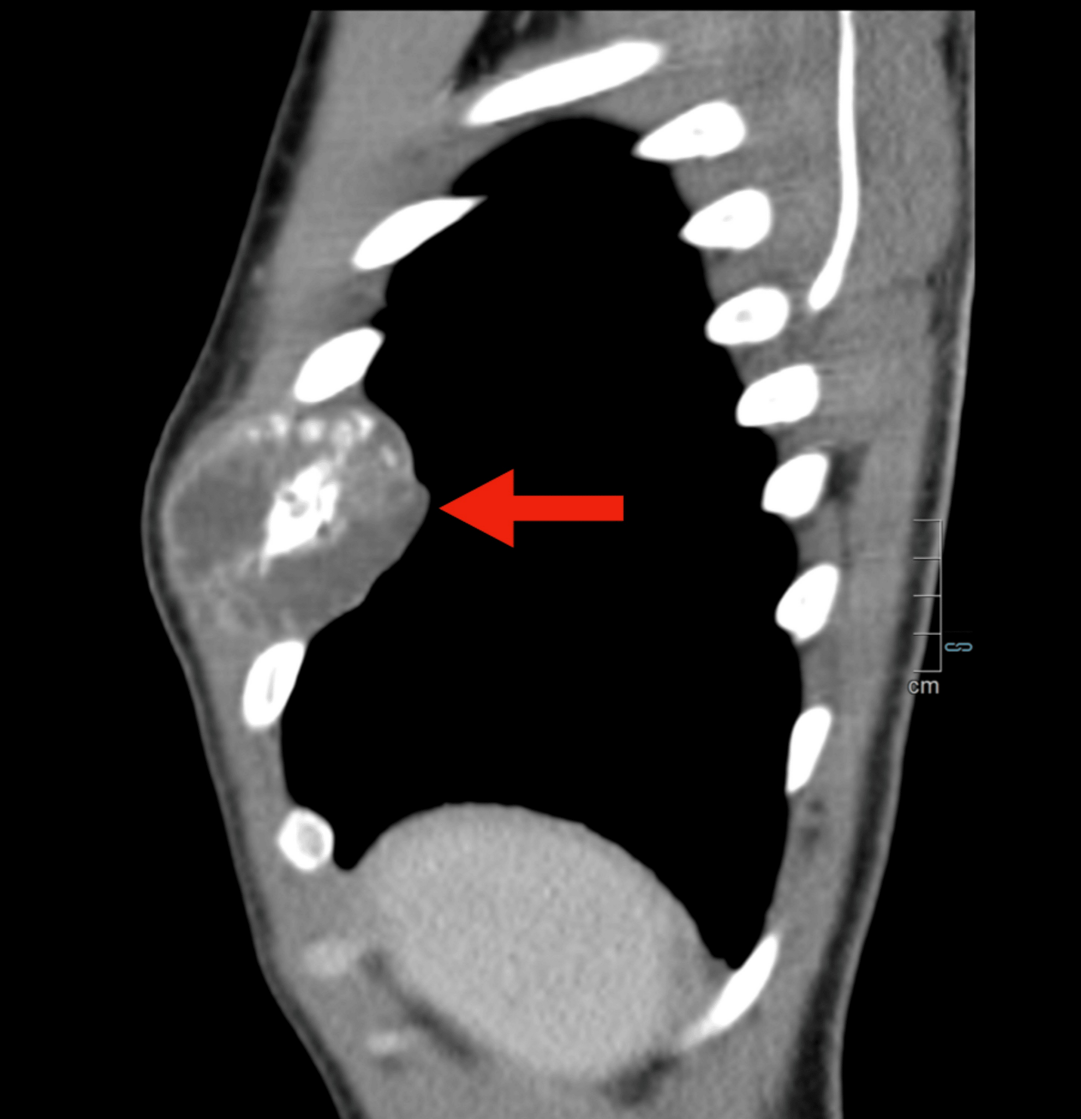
Sagittal contrast-enhanced CT scan of the thorax Voluminous soft-tissue lytic neoformation infiltrating the anterior aspect of the right sixth rib. This lesion shows heterogeneous contrast enhancement and some peripheral calcifications (red arrow).
